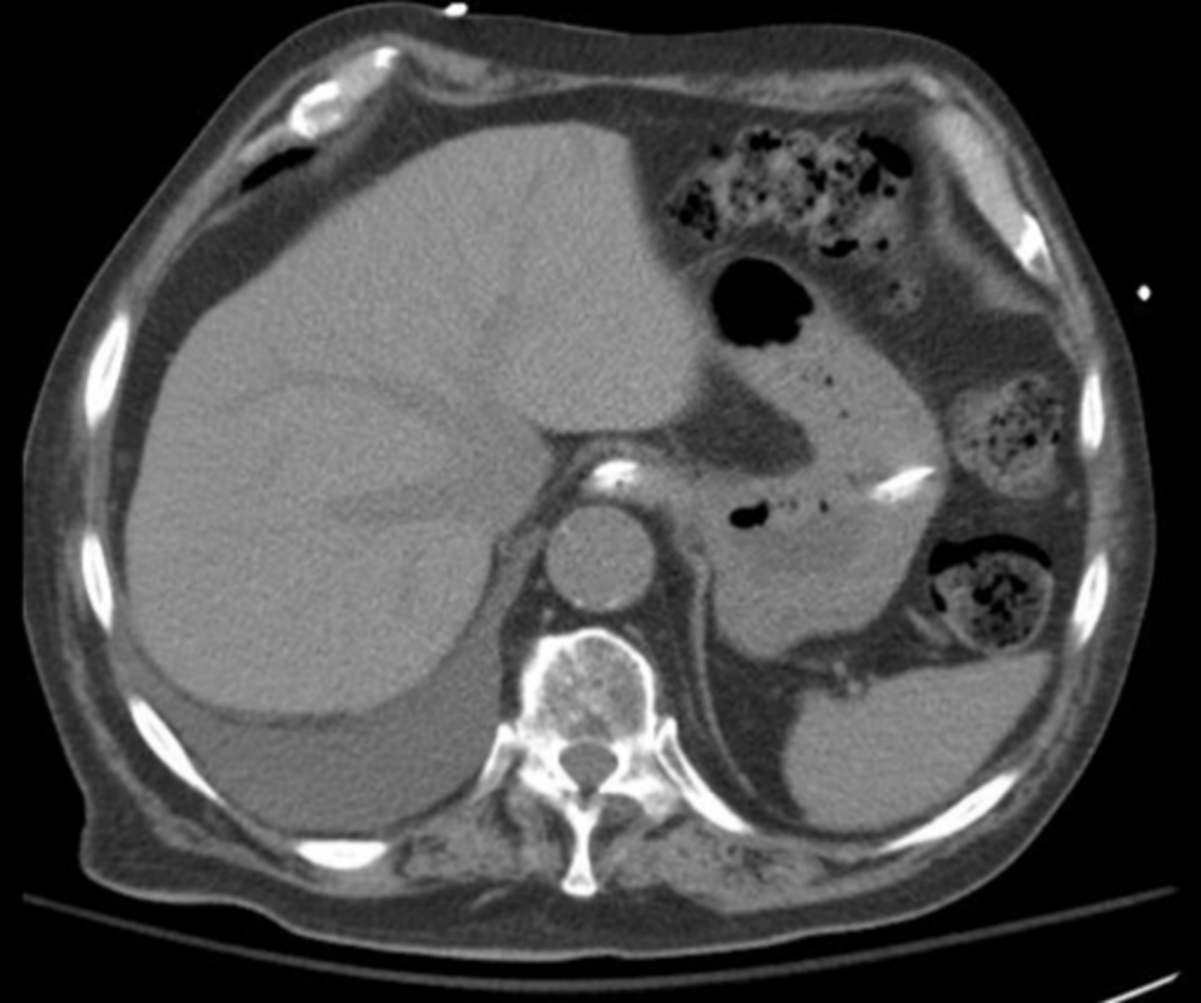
Computed tomography of abdomen showing normal liver echotexture.
